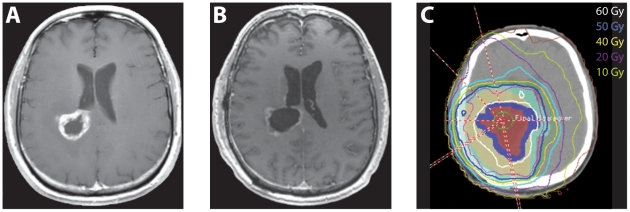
Diagnostic imaging and treatment plan from a representative case. A) Pre-operative contrast enhanced MRI. B) Post-operative contrast enhanced MRI showing resection cavity. C) Treatment planning CT with isodose lines depicting the dose distribution.
